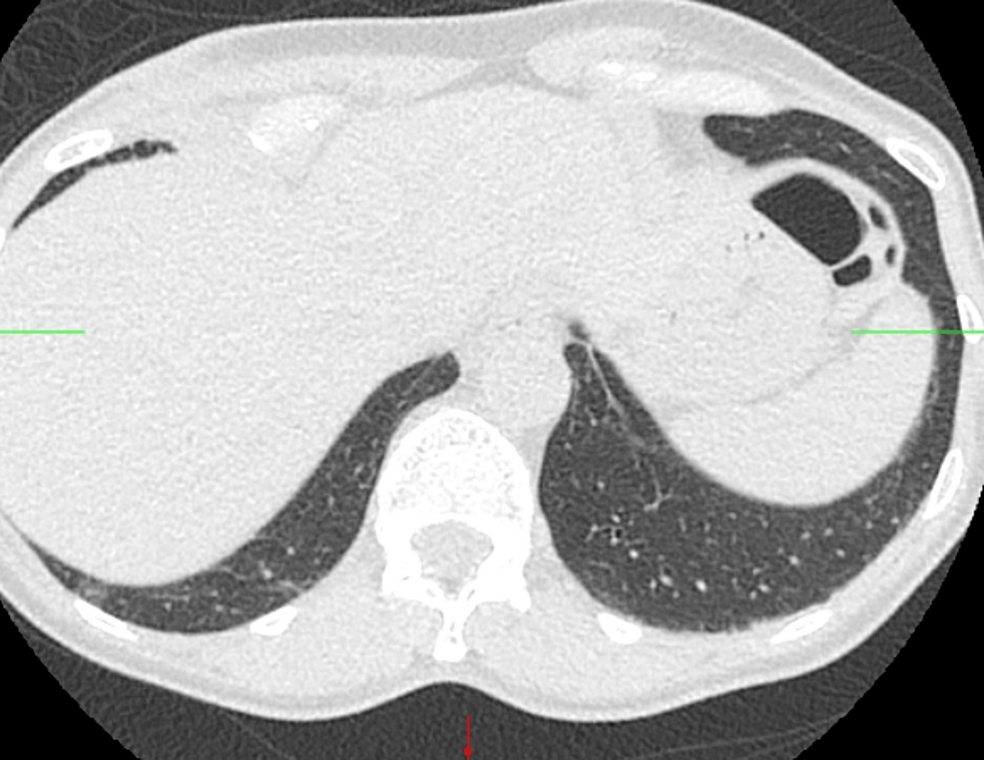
CT image 3 months post-surgery showing no residual nodules CT: computed tomography
